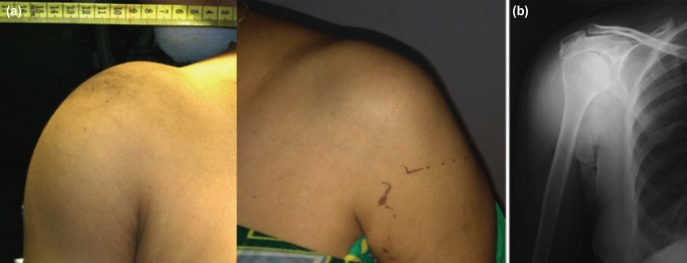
(a) Clinical photographs of sub-deltoid swelling. (b) Plain radiograph of right shoulder with normal glenohumeral articulation and sub-deltoid soft tissue shadow.
